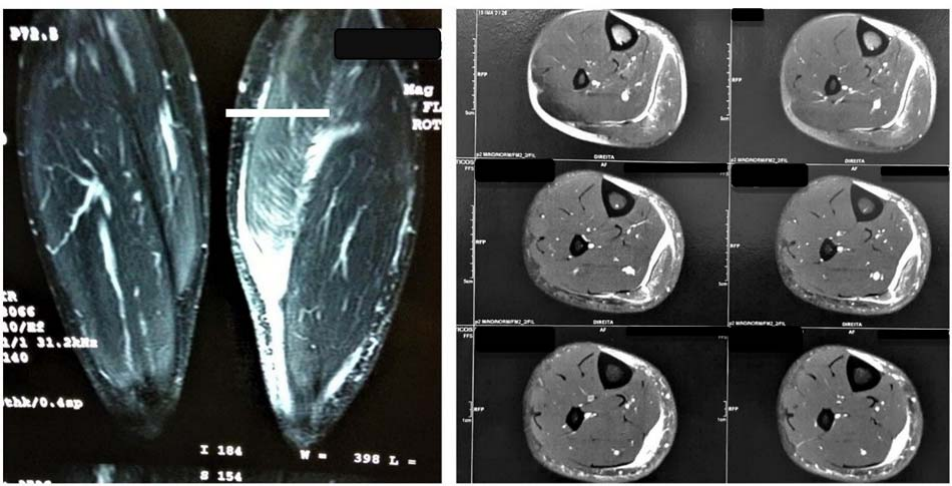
MRI images of Patient 1 and Patient 2, respectively. In the left image, a coronal section of the two legs weighted in a sensitive liquid sequence shows edema accompanied by a distal gap in the lesion topography. In the right image, we see multiple axial slices also showing fluid accumulation and muscle retraction in the musculotendinous transition.
